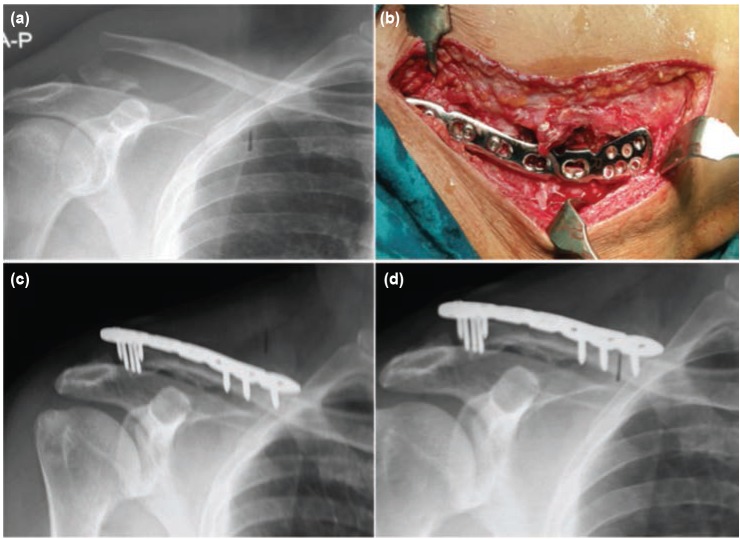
(a) Radiographs antero-posterior view of shoulder showing lateral end of clavicle fracture, (b) Intra-operative photograph after fixation with pre-contoured lateral third clavicular plate. Radiographs taken at (c) 6 months and (d) 9 months after surgery showing fracture union.
